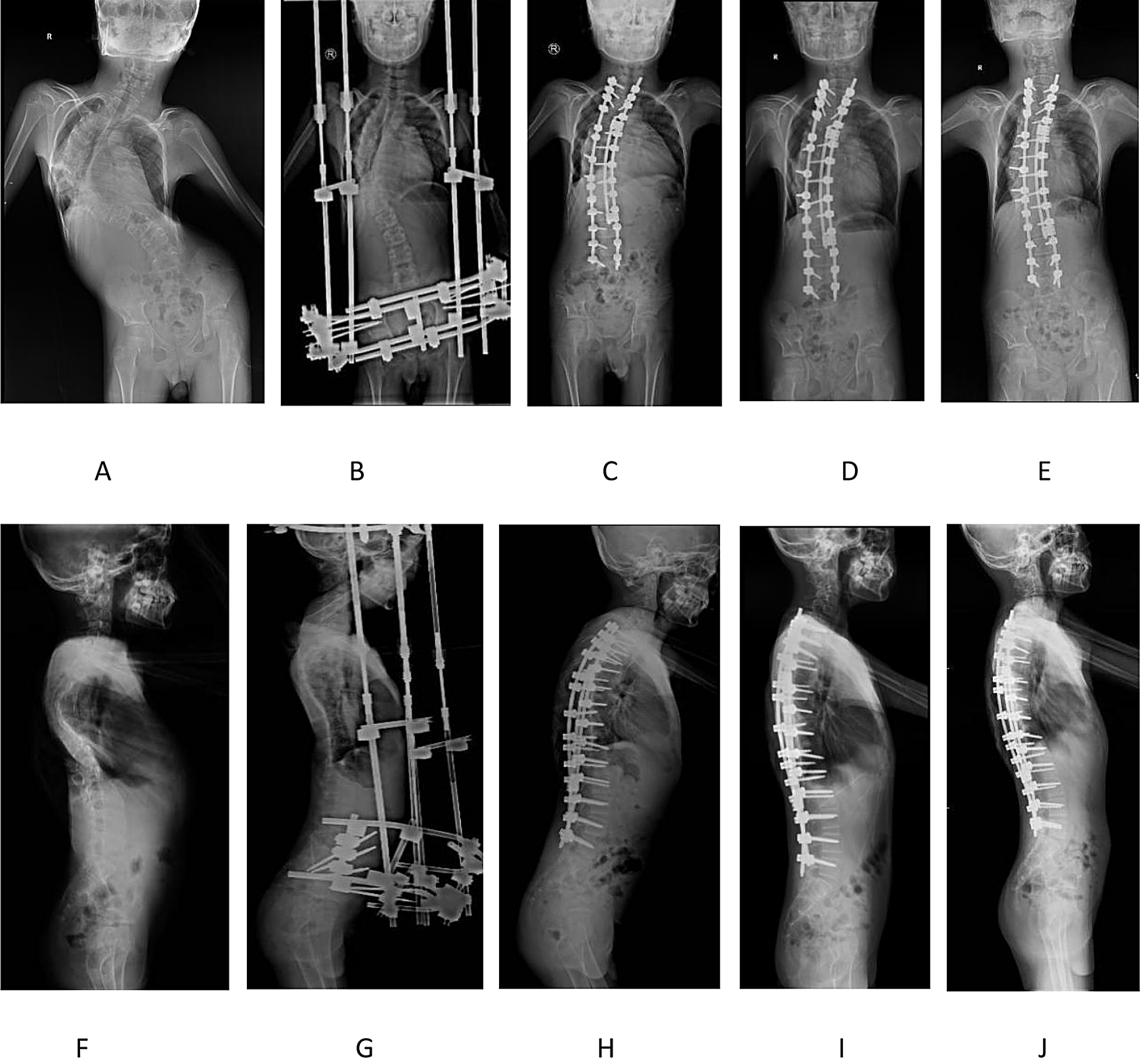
The appearance before and after treatment. (A) The anteroposterior view of spinal X-ray before surgery. (B) The anteroposterior view of spinal X-ray after traction. (C) The anteroposterior view of spinal X-ray 1 week after surgery. (D) The anteroposterior view of spinal X-ray 1 month after surgery. (E) The anteroposterior view of spinal X-ray 1 year after surgery. (F) The lateral view of spinal X-ray before surgery. (G) The lateral view of spinal X-ray after traction. (H) The lateral view of spinal X-ray 1 week after surgery. (I) The lateral view of spinal X-ray 1 month after surgery. (J) The lateral view of spinal X-ray 1 year after surgery
